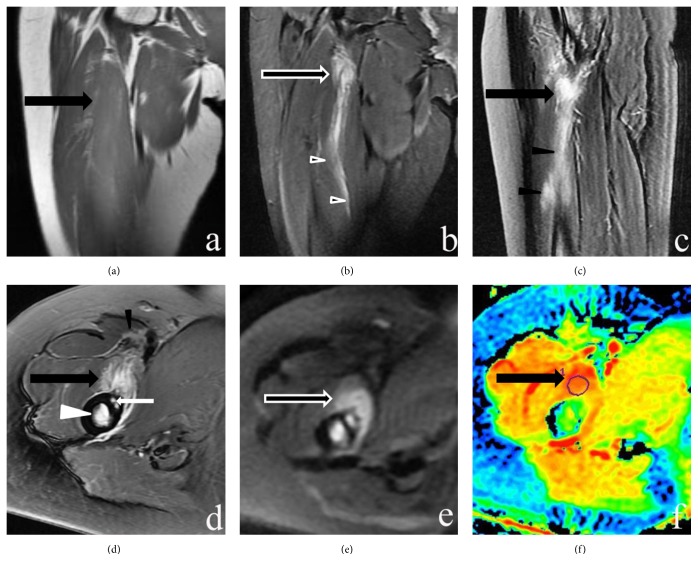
MR imaging of the right thigh. (a) Coronal T1-weighted image shows an ill-defined isointense lesion in the iliopsoas muscle (large black arrow). (b-d) Sagittal, coronal, and axial fat-suppressed T2-weighted images reveal a hyperintense lesion with a “striate pattern” in the iliopsoas muscle (large black arrow) with a pattern of edema in the iliopsoas and sartorius muscles (black arrowheads). The femur bone shows bone marrow edema (white arrowhead). The femur periosteum displays patchy areas of hyperintensity in FSE T2WI images (small white arrow). (e and f) Axial DWI and ADC mapping display hyperintensity in DWI and high ADC values of the lesion, which indicate a T2 shine-through effect.
